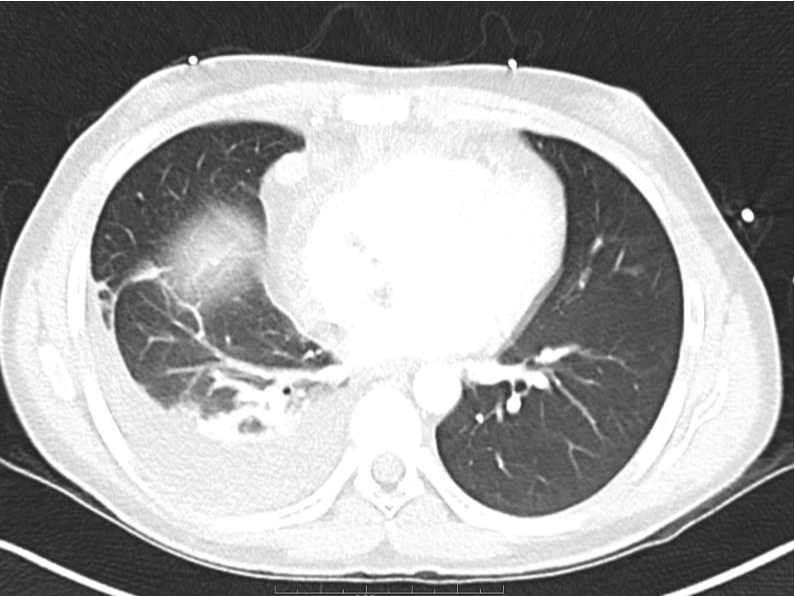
CT chest with angiography: interval increase in anterosuperior mediastinal mass to 12.3 × 9.9 cm with SVC compression; progressive pericardial, moderate right effusion, and lower lobe consolidation [5].
